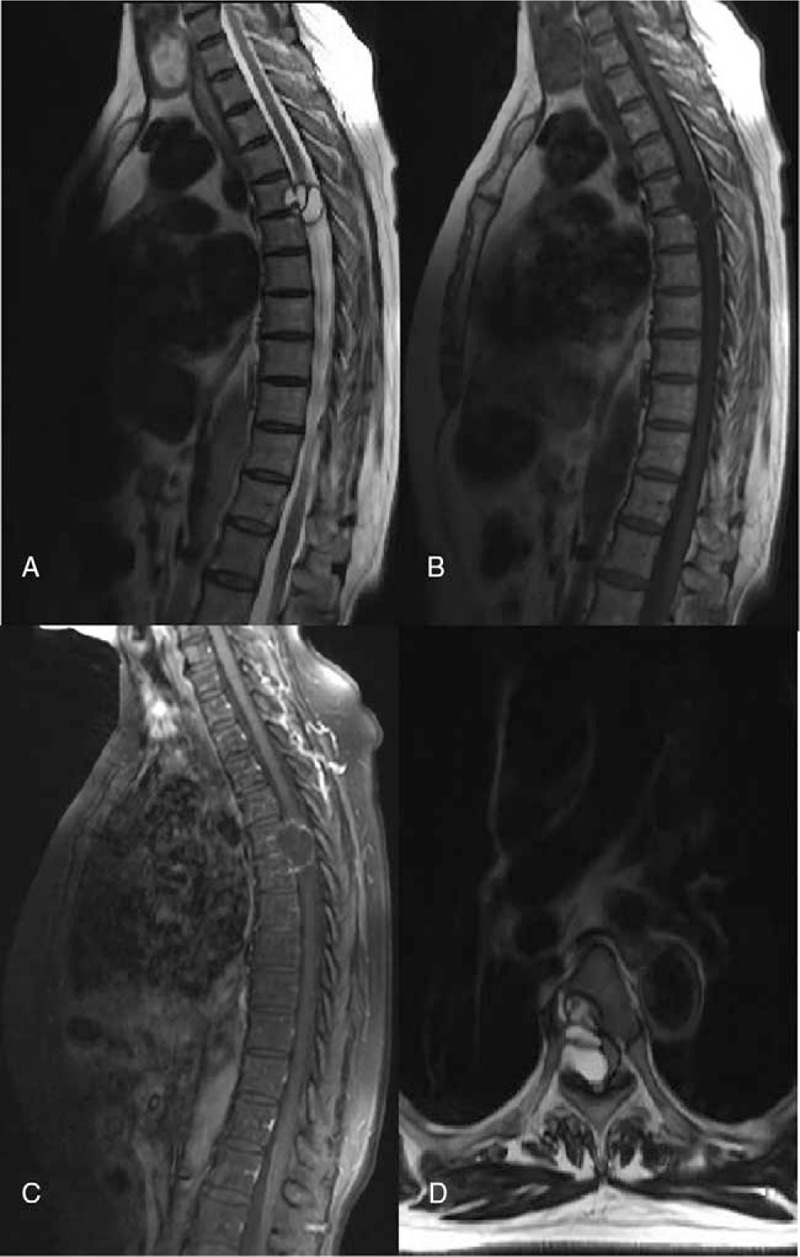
Preoperative MR images. (A and B) Sagittal T2/T1-weighted image showing that a cystic lesion with septations has been embedded in T5; (C) Sagittal enhanced T1-weighted image showing the cystic lesion wall and enhanced septa; (D) Axial T2-weighted image showing compression of the spinal cord by the extradural cystic lesion.
